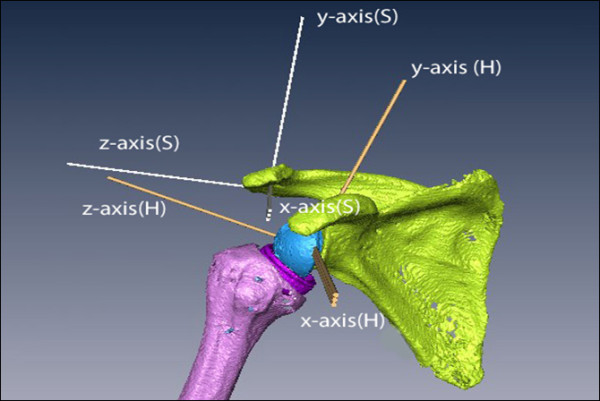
Three-Dimensional shoulder model created from CT-scans after implantation of a polycarbonate-resin inverse shoulder prosthesis. Two coordinate systems (Scapula (S); Humerus (H)) were defined according to the recommendations of the Society of Biomechanics.
